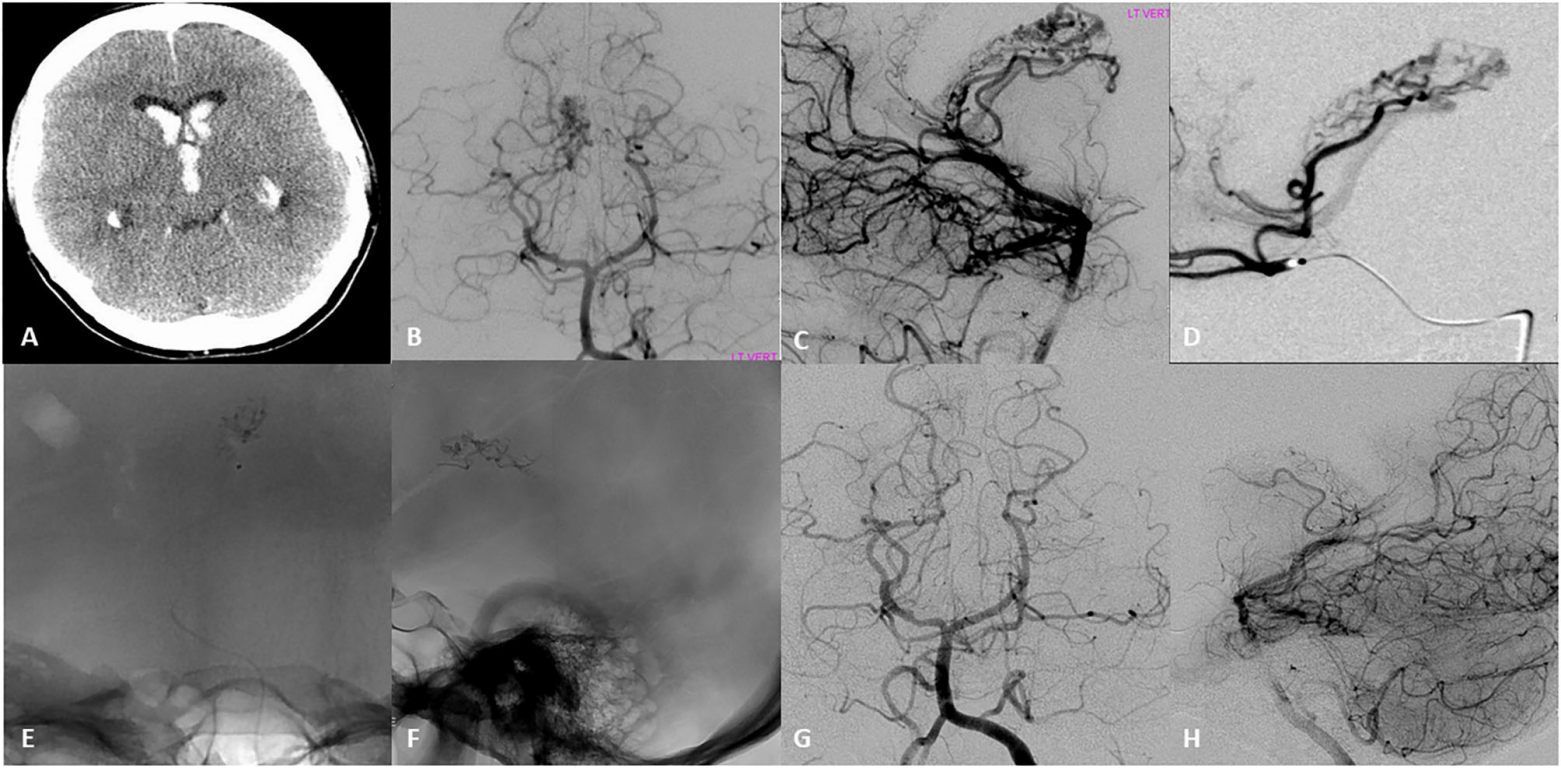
Seventeen-year-old right-handed healthy girl that presented with initial headache nausea and vomiting, that progress to decrease level of consciousness within hours. (A) Initial head CT revealed intraventricular hemorrhage in the lateral ventricles and third ventricle, for which she underwent emergent ventriculostomy. (B,C) Initial DSA AP and lateral left vertebral artery injection revealed an arteriovenous malformation with a compact nidus located in the lateral ventricle. Arterial feeders arise from the post-erolateral and post-eromedial choroidal arteries, both branches of the right posterior cerebral artery. The drainage occurred into the right internal cerebral vein. (D) Superselective DSA examination showed a medial posterior choroidal artery that appeared to feed the nidus of the AVM with opacification of the right internal cerebral vein. (E,F) AP and Lateral native images demonstrate the N-Butyl cyanoacrylate (NBCA) cast into the nidus after microcatheter embolization of two pedicles. (G,H) DSA Ap and lateral 6–month follow up showed with complete obliteration of the AVM.
